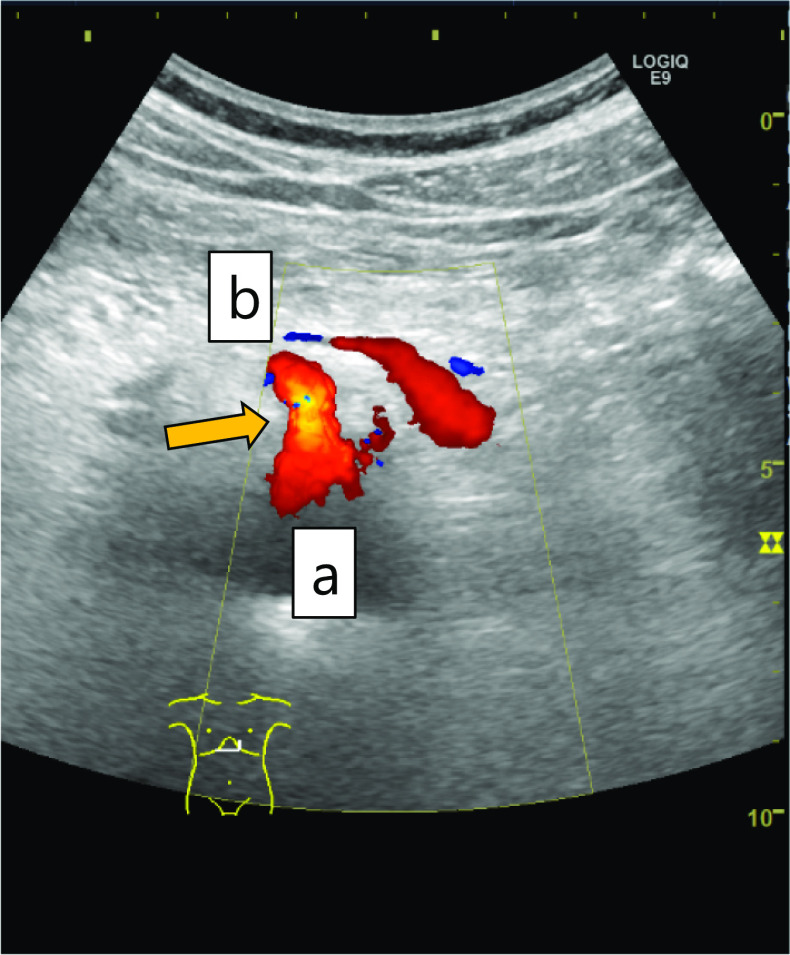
Postoperative ultrasonography. No stenosis was seen at the celiac artery origin: a aorta, b celiac artery
